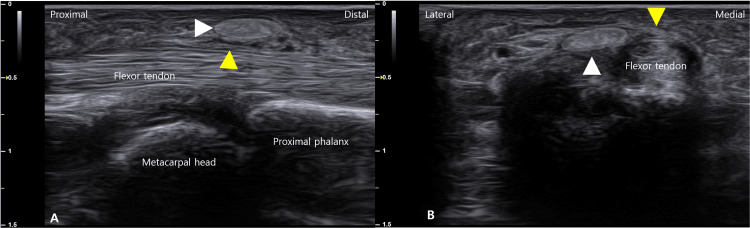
Long- and short-axis ultrasonography of the A1 pulley. In the long-axis view, a hyperechoic calcific deposit (white arrowhead, white-shaded area) is visualized above the A1 pulley (yellow arrowhead) (A), and in the short-axis view, the calcific focus (white arrowhead, white shaded area) is observed on the lateral side of the A1 pulley (yellow arrowhead) (B).
